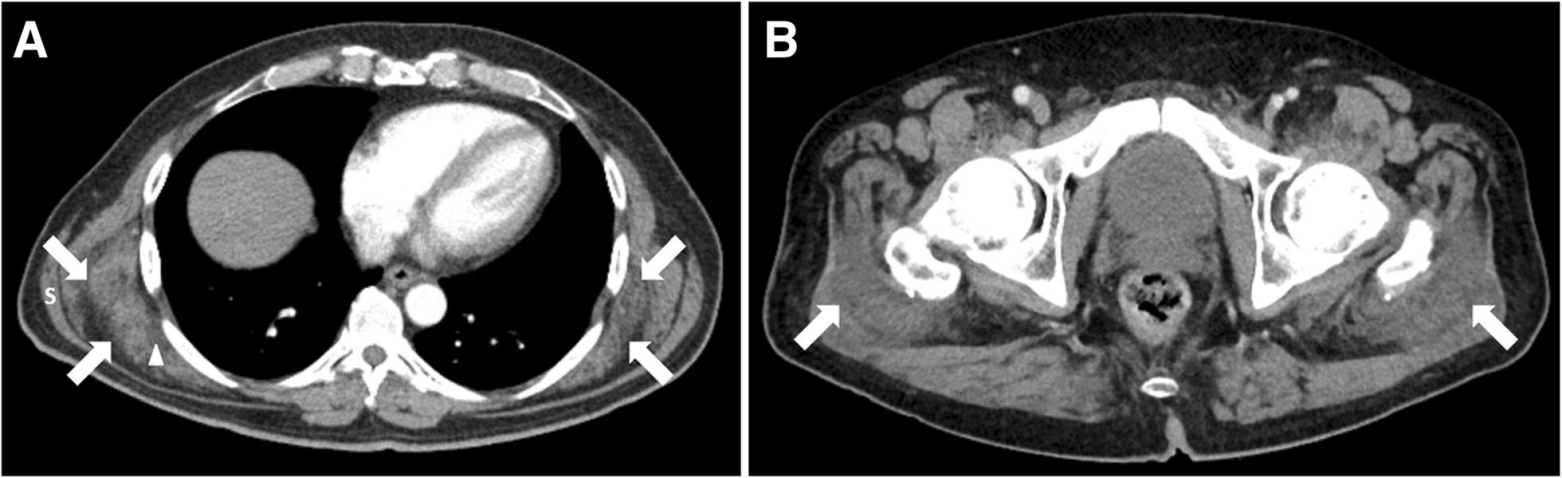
Axial contrast-enhanced CT images showing bilateral well-defined unencapsulated masses within the infrascapular regions (arrows in a), deep to the serratus anterior muscles (S) with attenuation similar to that of the adjacent skeletal muscle and internal areas of streaky fat (arrowhead). In addition, there are similar appearing bilateral poorly defined soft tissue masses (arrows in b) at the level of the greater trochanters
